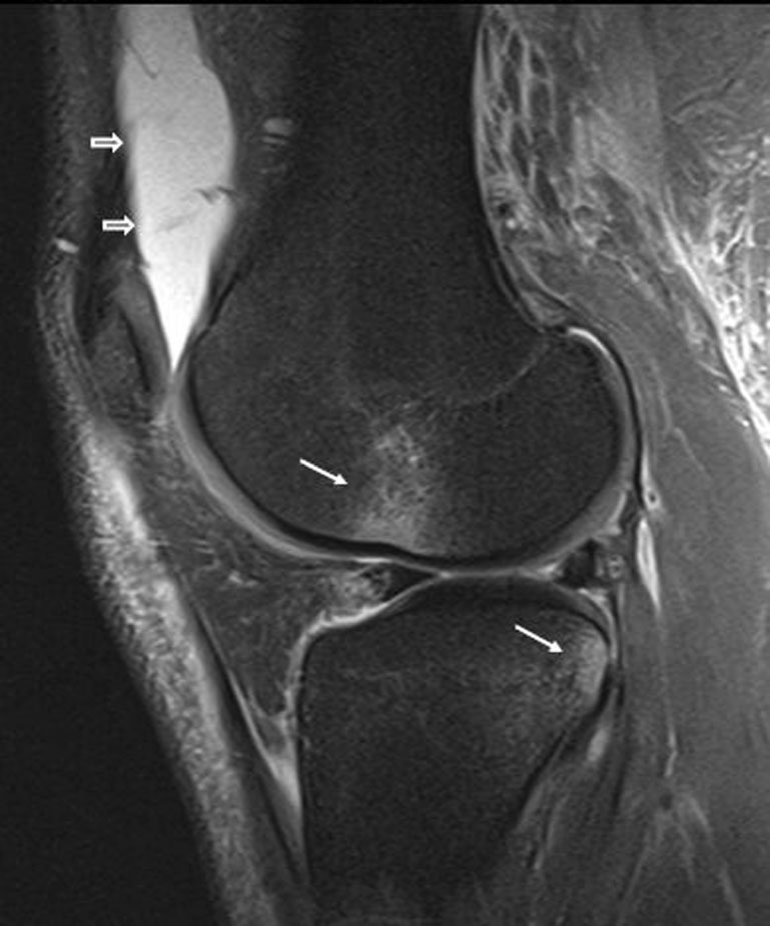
HFP acute trauma (pivot shift). MRI proton density with fat saturation image shows HFP apex oedema (*thin arrows*), oedema of the external femoral condyle and of the corresponding tibial plateau (*thin arrows*), with joint effusion (*wide arrows*)
